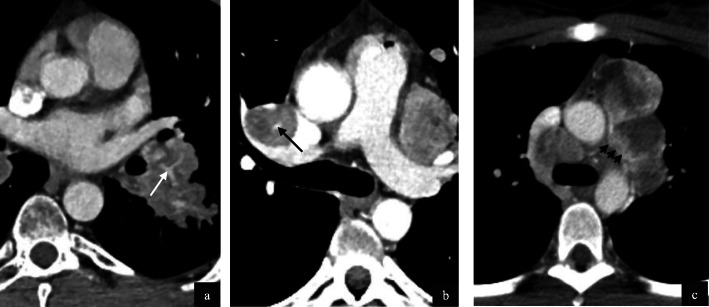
Contrast enhanced axial CT in three patients with nodal metastases from primary ASPS (a-c). Left hilar nodal tissue in a 31 year old male patient demonstrates high attenuation with prominent central vessels (a, white arrow). Feeding vessels are demonstrated alongside and within nodal disease in a 37 year old male patient (b, black arrow). Metastatic mediastinal nodal disease in a 28 year old female (c) demonstrates marked central necrosis, however peripheral hypervascularity is still appreciated (c, black arrowheads)
